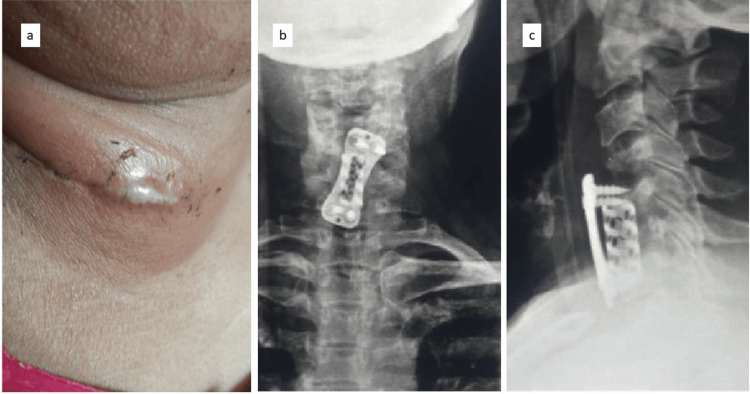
Clinical and radiographic picture of the patient at three weeks postoperatively. (a) Discharging sinus at the surgical site; (b) anteroposterior radiograph at three weeks postoperatively; (c) lateral radiograph at three weeks postoperatively
